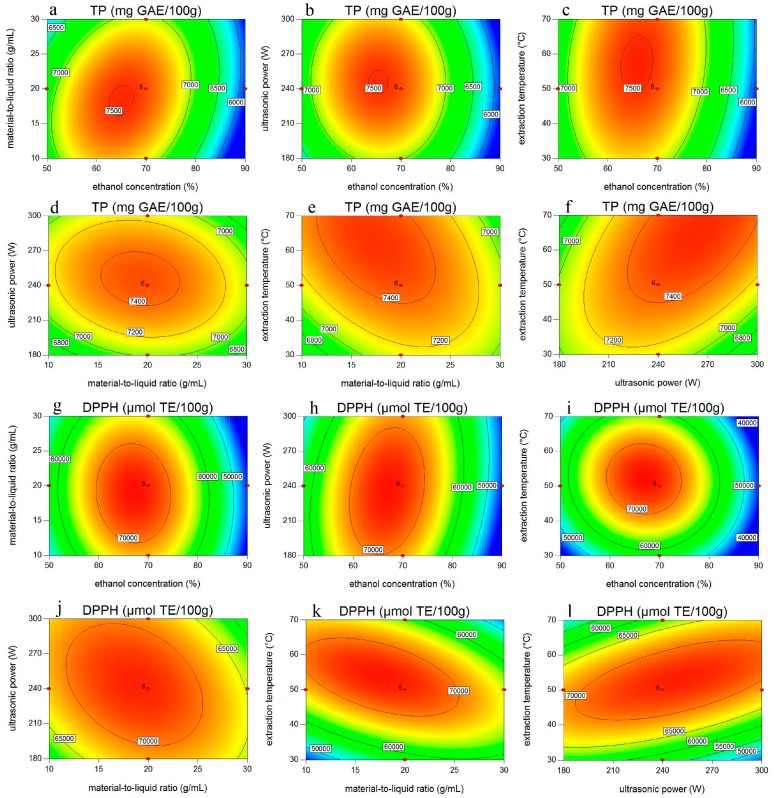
Contour plots showing the effects of four variables (solvent concentration, material to liquid ratio, ultrasonic power, and extraction temperature) and their interactions on extraction efficiency of total phenols (TP, a–f) and their DPPH• scavenging capacity (g–l). The other two variables in each of the Figures were kept constant at their respective central experimental values.
